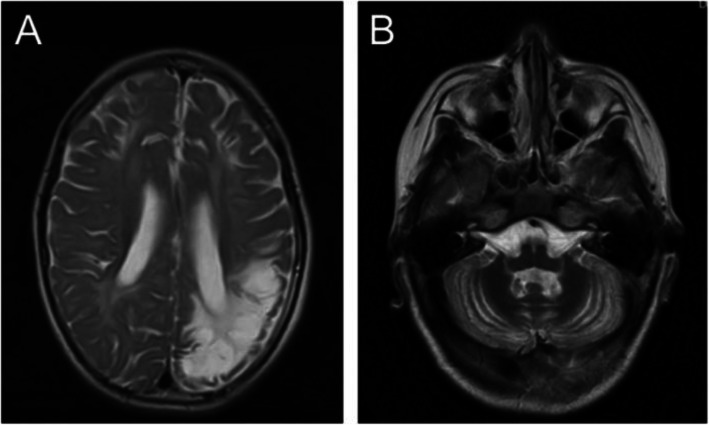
Enhanced Brain MRI of a 6 years and 11 months old male patient. a MRI image of brain scan showed encephalomalacia and laminar necrosis with diffuse white matter disorder. b MRI scan of the brain demonstrated cerebellar atrophy in the patient
